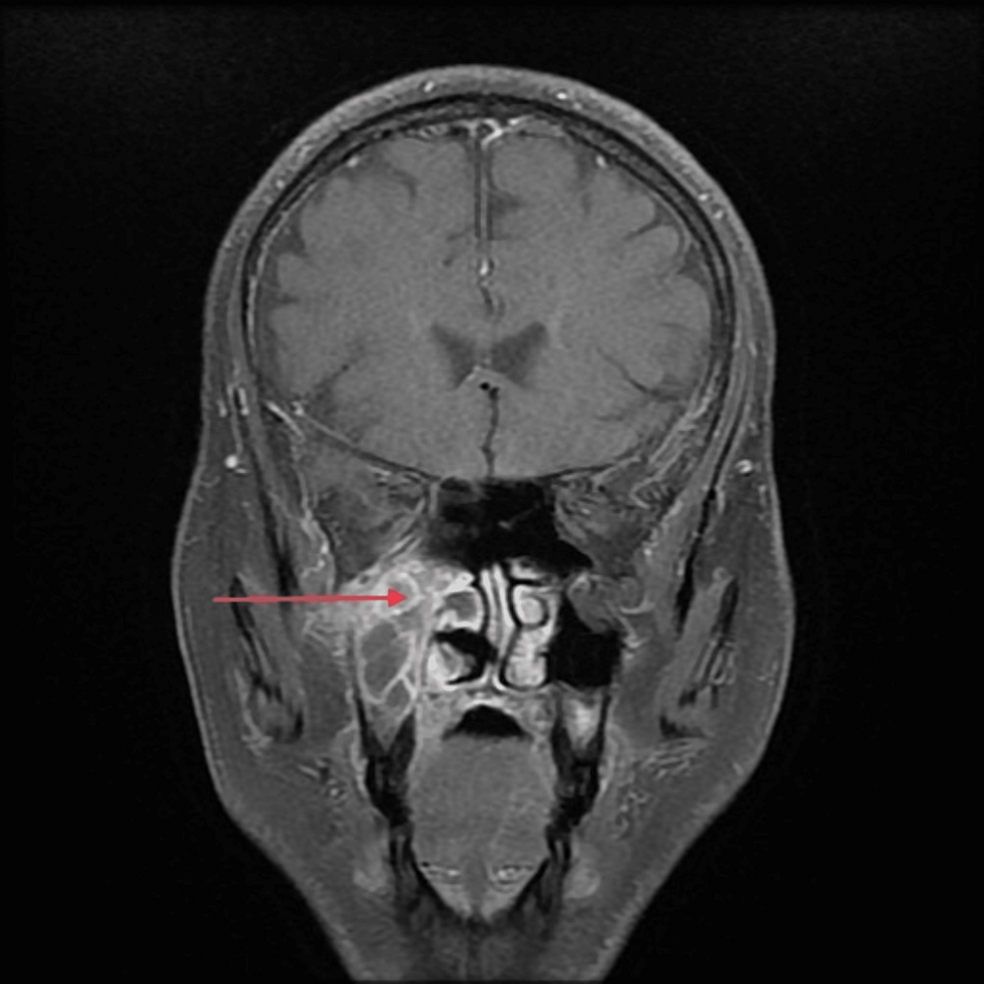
T1-weighted MRI head (coronal section) of Case Five showing right maxillary sinusitis and infiltration (red arrow)
